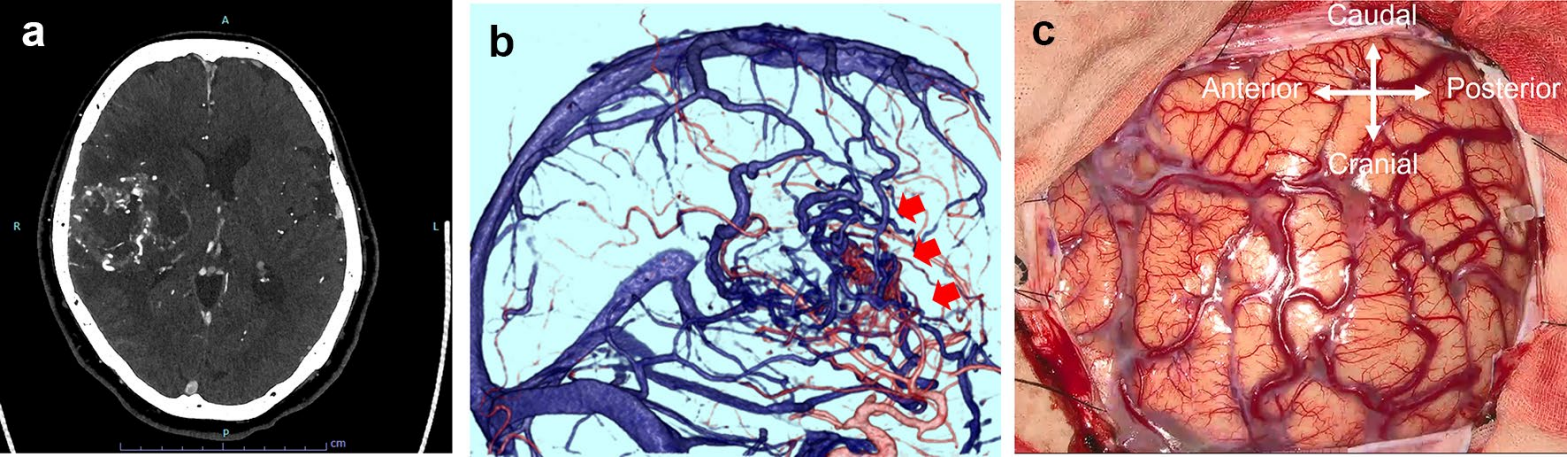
Glioblastoma. The case is of a woman in her 70 s. A few months prior, she fell and bruised her knee. Since then, she experienced difficulties in walking, and because of the progression of her symptoms, she visited a hospital. PCD-CTA (axial image: kernel Hv60) showed a ring-enhanced mass in the right fronto-parieto-temporal lobe (a). There were numerous microvessels in the wall of the mass, suggesting a hypervascular tumor with early venous filling and an AV shunt (a, b). Glioblastoma was suspected, and surgery was performed. Intraoperative view reveals a red cortical vein (c). *PCD-CTA* photon-counting detector-computed tomography angiography, *AV* arteriovenous
